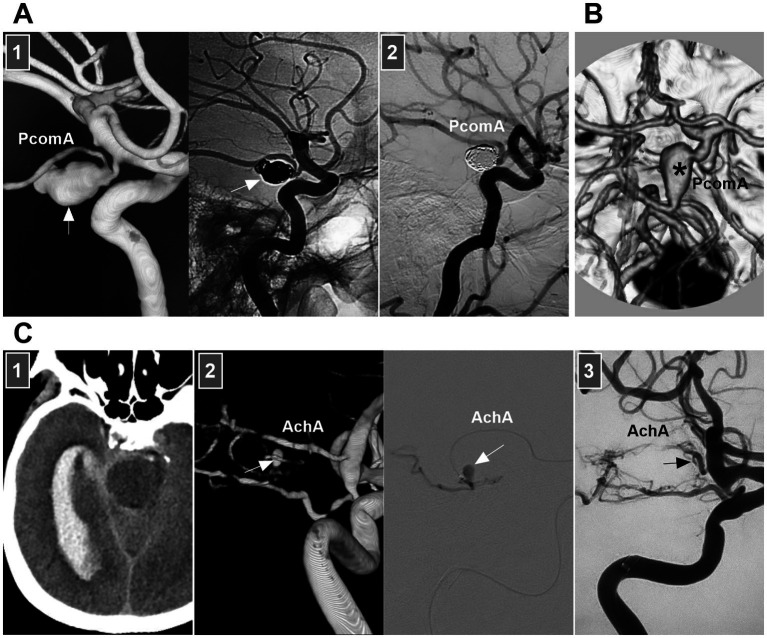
EVT of PcomA and AchA dissections. (A) Panel 1: DSA (left panel) showing a PcomA trunk dissecting aneurysm (arrow), unsubtracted DSA (right panel) showing that the aneurysm was coiled (arrow), the PcomA was preserved. Panel 2: Four-year follow-up DSA showing the aneurysm had no recurrence. (B) CTA showing a PcomA trunk dissecting aneurysm (asterisk), the lesion can be treated by parent artery occlusion. (C) Panel 1: CT showing subarachnoid hemorrhage. Panel 2: DSA (left panel) and microcatheter angiography (right panel) showing a dissecting aneurysm (arrow) at distal AchA. Panel 3: DSA showing that the dissection was occluded by casting liquid embolic agent and the proximal AchA trunk (arrow) was preserved. AchA, anterior choroidal artery; CT, computed tomography; CTA, CT angiography; DSA, digital subtraction angiography; EVT, endovascular treatment; PcomA, posterior communicating artery.
